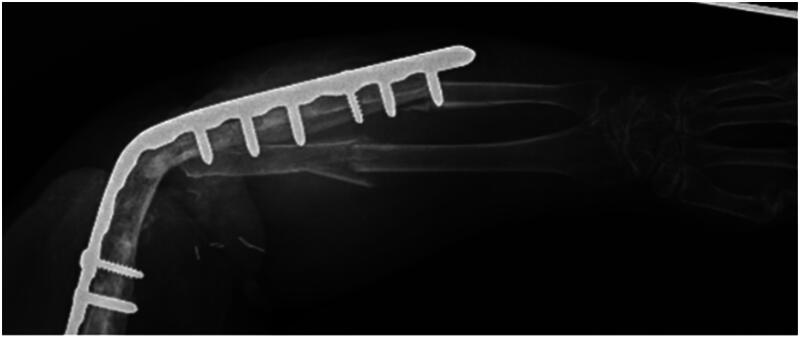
X-Ray taken prior to surgery. Midshaft fracture of the radius and ulna involving the elbow arthrodesis.
